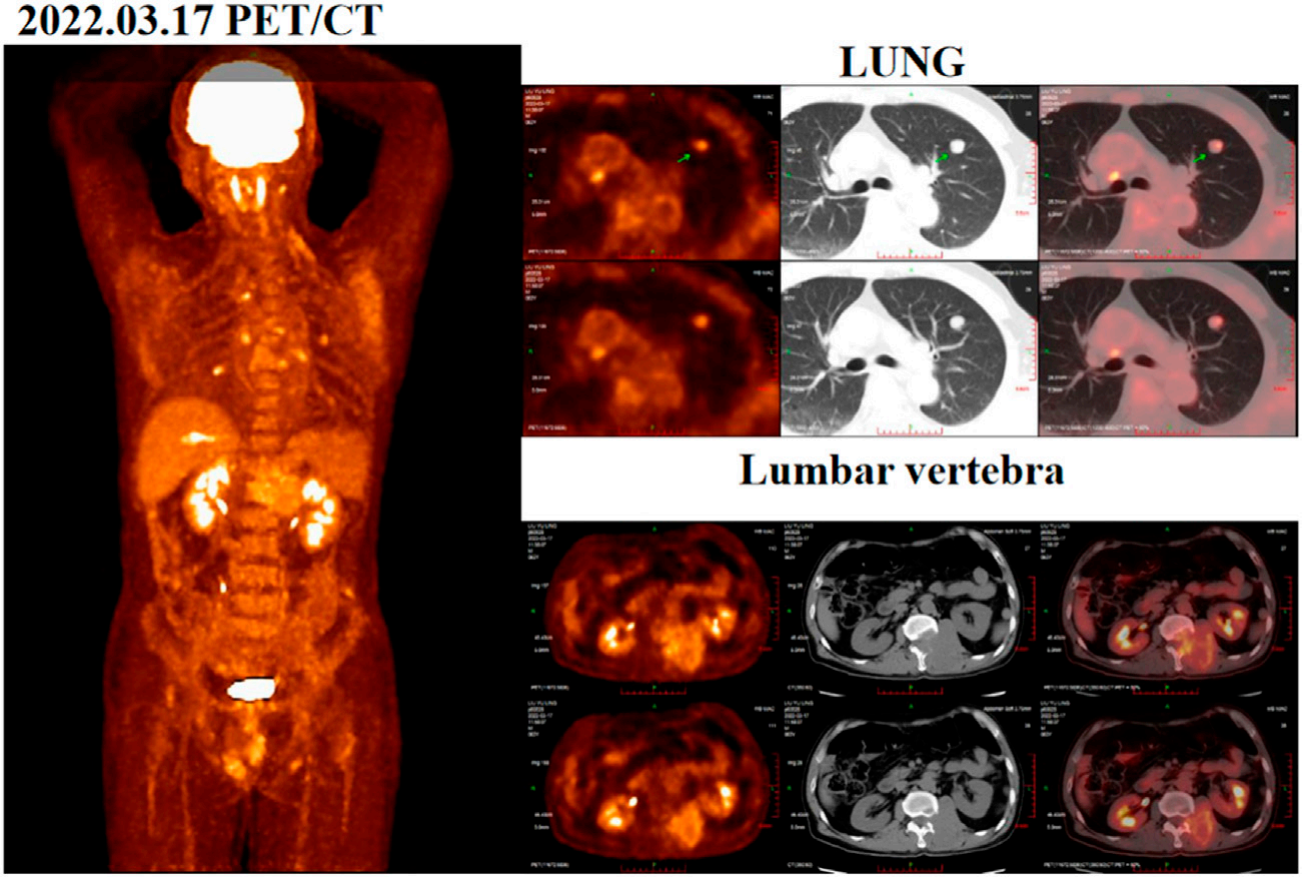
Positron emission tomography/computed tomography (PET/CT) images acquired before surgery on 17 March 2022.
